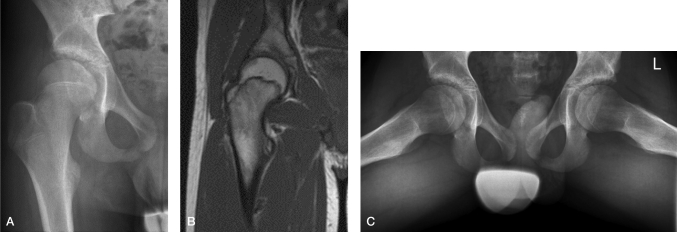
Case 1. The typical coxa valga due to coalescence (arrow) on a standard AP view (A) and T1-weighted coronal MRI of the pelvis during growth at the age of 13 years (B). On the Lauenstein view, the head-neck offset is small (C).
